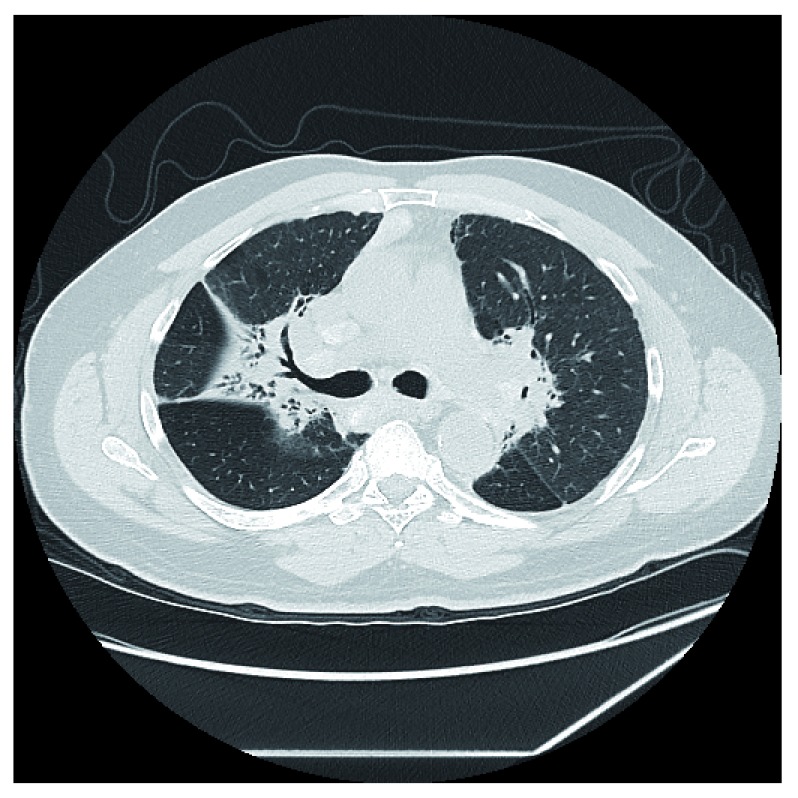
Chest computed tomography image of a patient with fibrotic pulmonary sarcoidosis. Note how the fibrosis is concentrated centrally along the bronchovascular bundles. This is the same location where the granulomas from pulmonary sarcoidosis tend to deposit. This location of fibrosis provides anatomic evidence that the fibrosis in sarcoidosis is a by-product of the granulomatous inflammation of the disease. We confirm that we have obtained permission to use this image from the patient included in this presentation.
